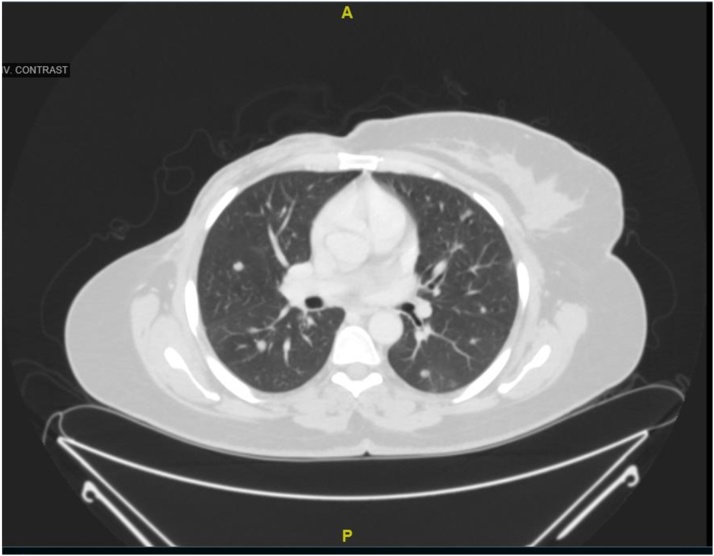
CT-Scan chest demonstrating bilateral multiple pulmonary nodules (Post-operative).
